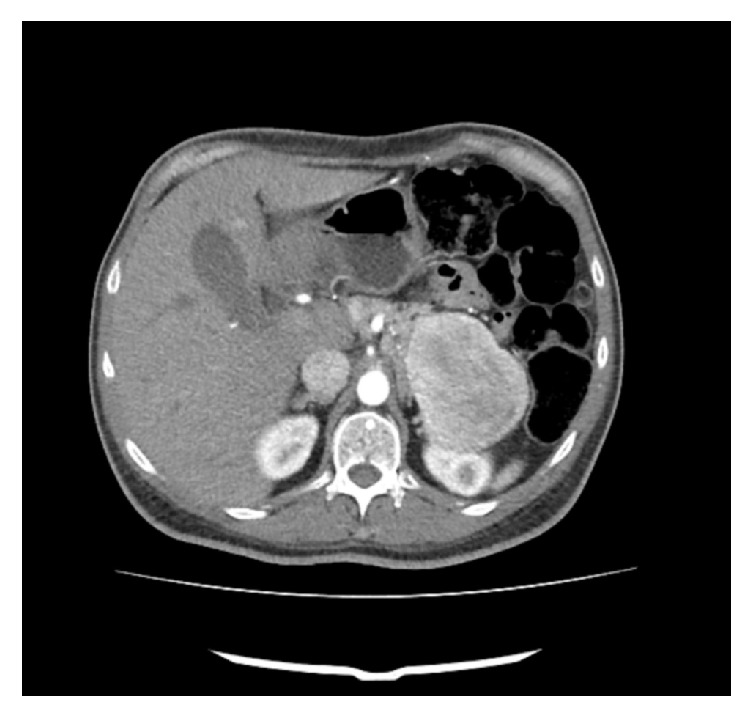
(CT) tail of pancreas with hypervascular heterogeneous and well-defined mass with lobulated edges measuring 6.8 × 7.7 × 6.4 cm.
